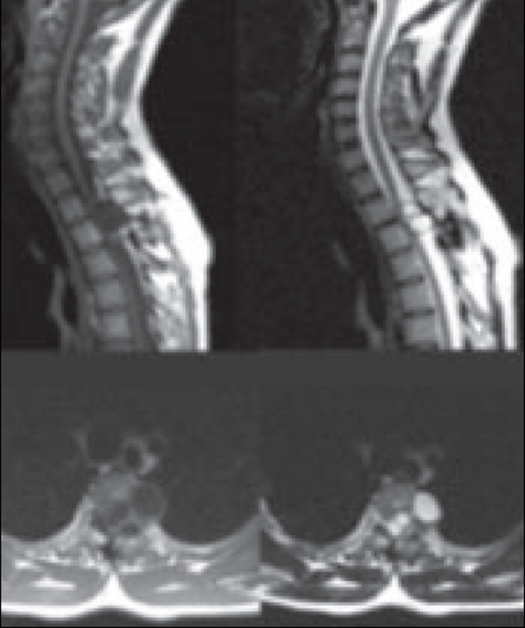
T1W- and T2W- sagittal (above) and axial (below) MRI images showing spinal cord compression
